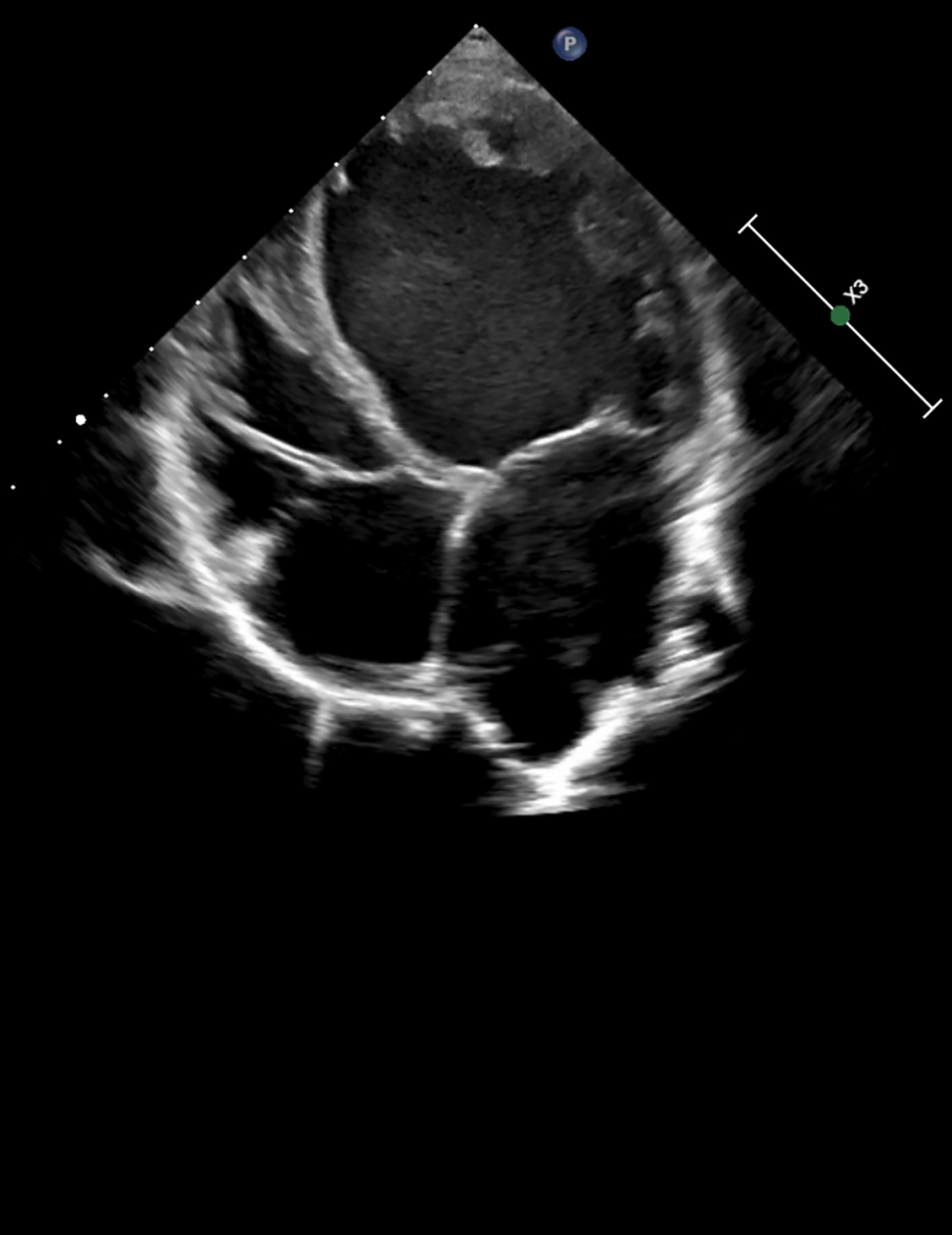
Echocardiographic image in a parasternal long-axis view showing the left ventricle, left atrium, and aortic root. Cardiac structures appear with clear wall delineation, suggesting preserved image quality for structural assessment.
